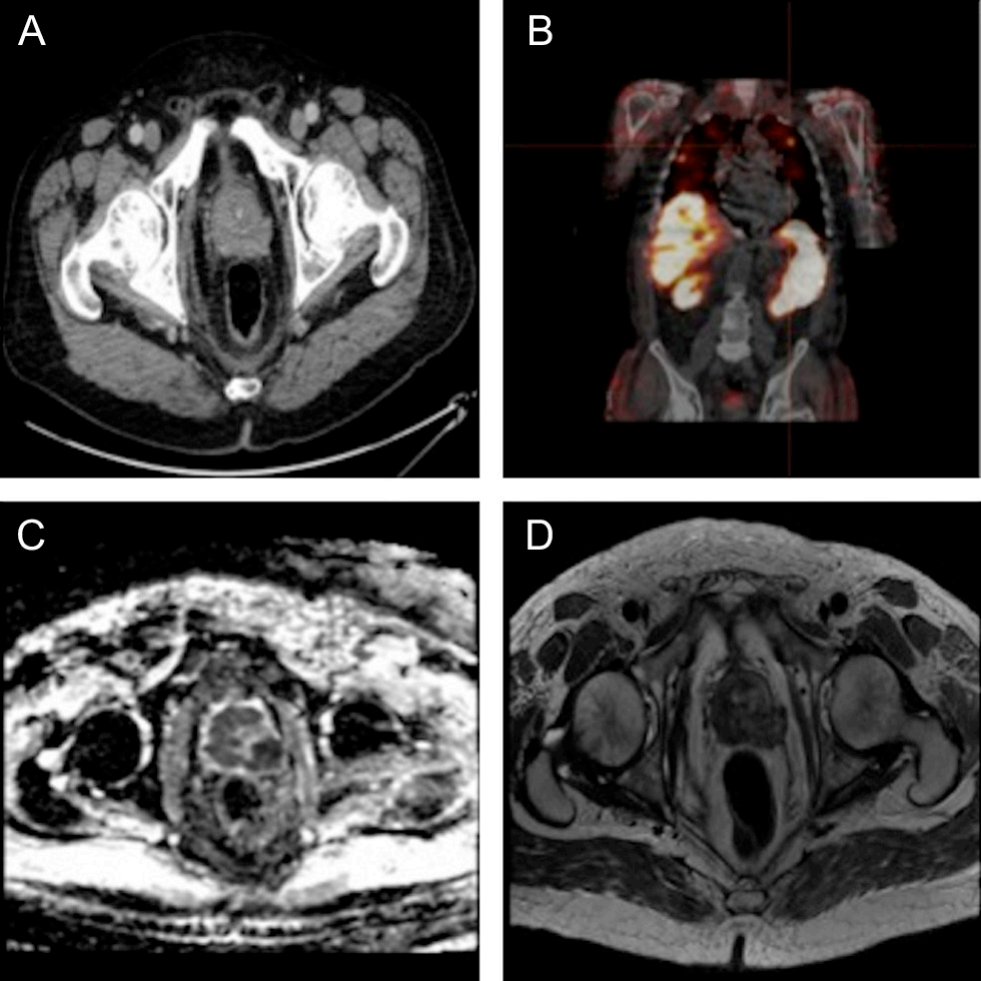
(A) CT imaging showing irregular prostate margins with possible extracapular spread. (B) Octreotide SPECT showing the presence of octreotide-avid pulmonary metastases. (C) Diffusion-weighted MRI imaging with ADC at 550 mm^2^/s. (D) T2-weighted MRI showing extensive signal abnormality of the prostate with extracapsular spread.
